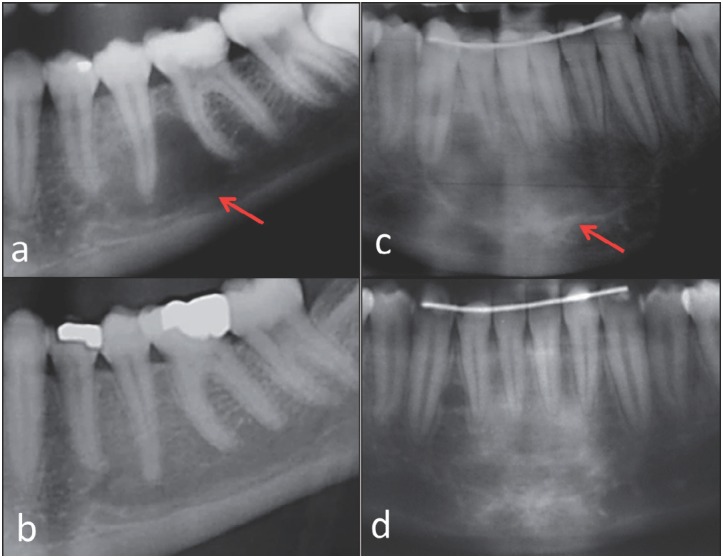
Radiographs showing spontaneous resolution of idiopathic bone cavity (IBC). a: A 15-year old female displaying an unilocular, well-defined radiolucency in the posterior mandible (red arrow); b: Final panoramic radiograph of the same patient after eight years of follow-up, showing complete resolution of the lesion; c: A 15-year-old female displaying an unilocular, well-defined radiolucency with scalloped margin surrounding the teeth apex in the anterior mandible (red arrow); d: After eight years of follow-up, we observed regression of the lesion
